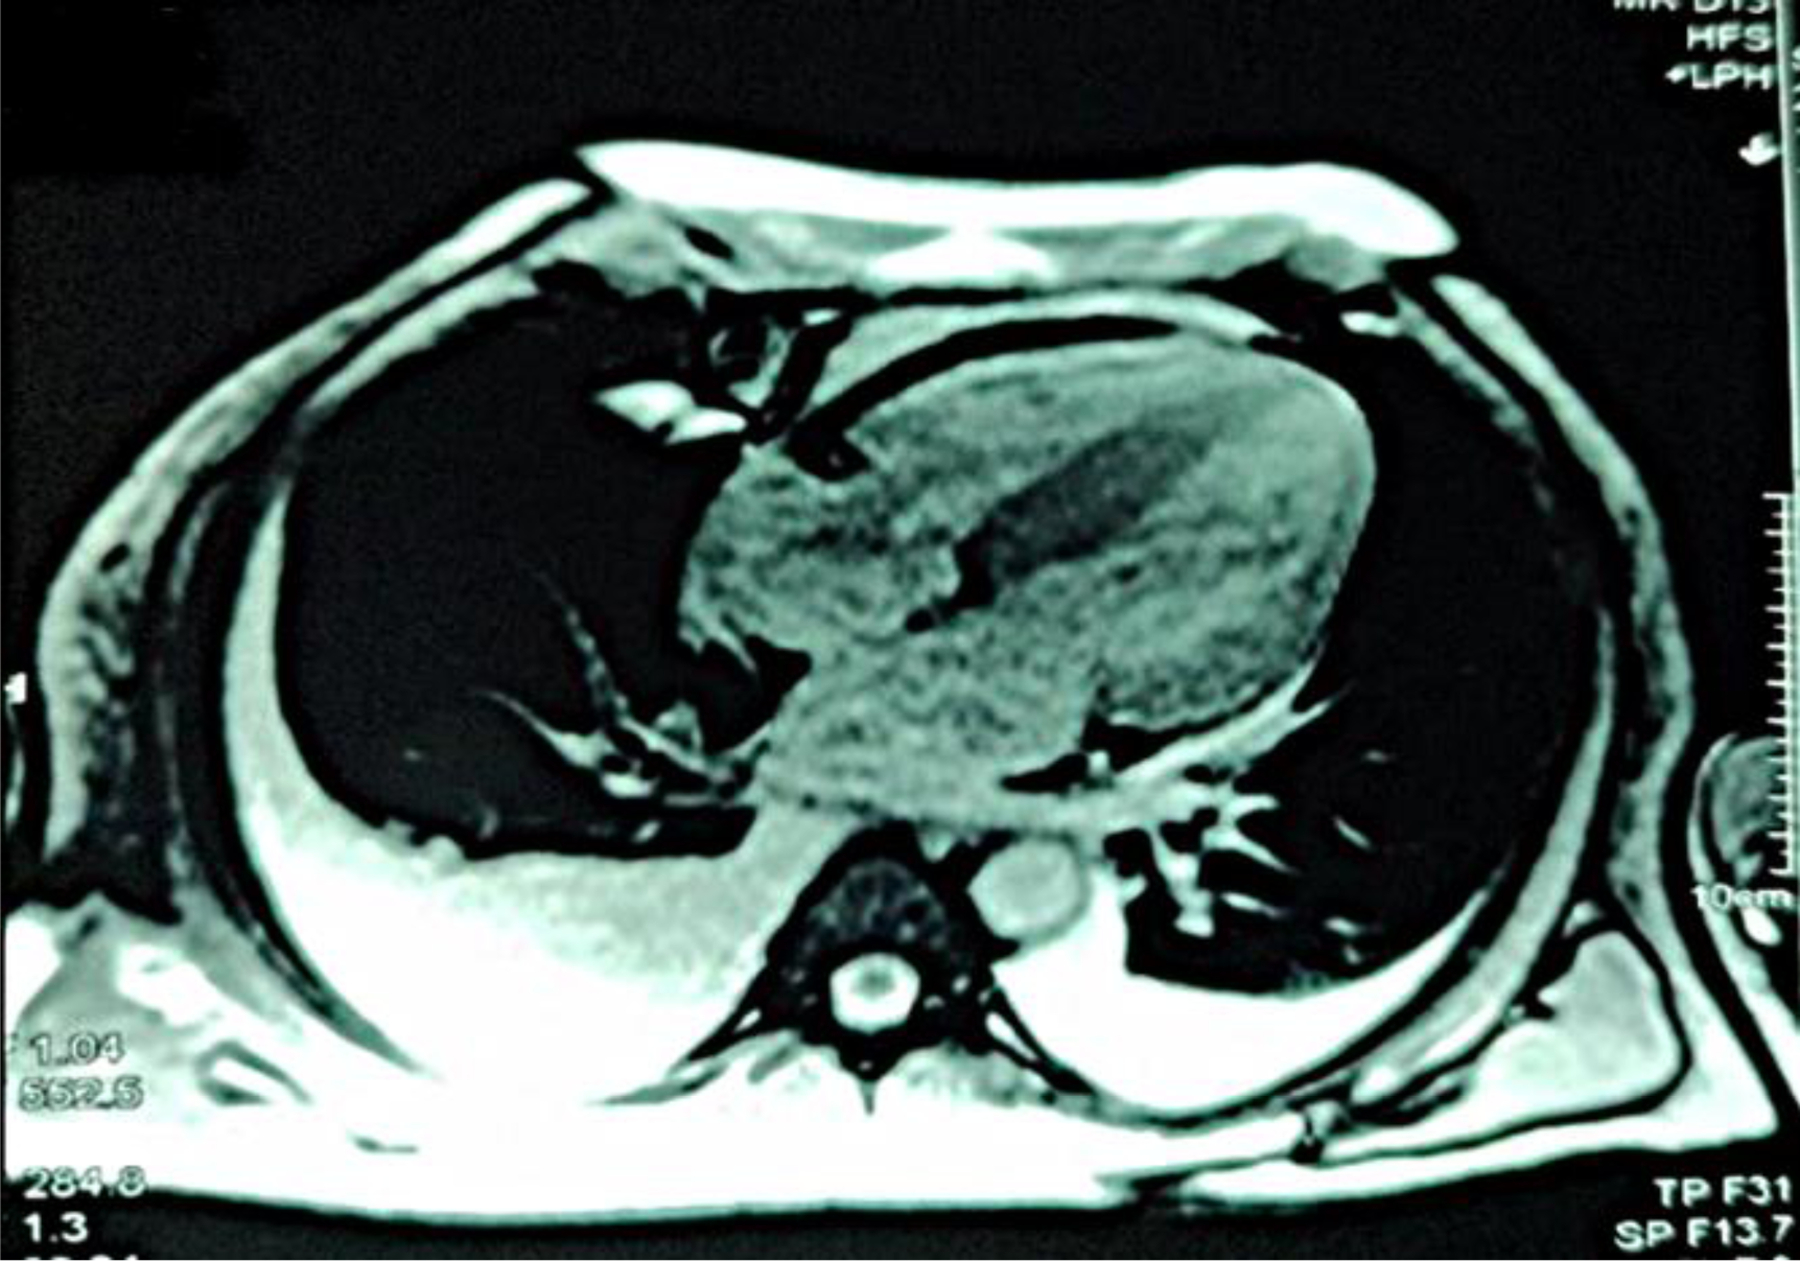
Diffuse global late gadolinium enhancement observed on cardiac magnetic resonance imaging.
